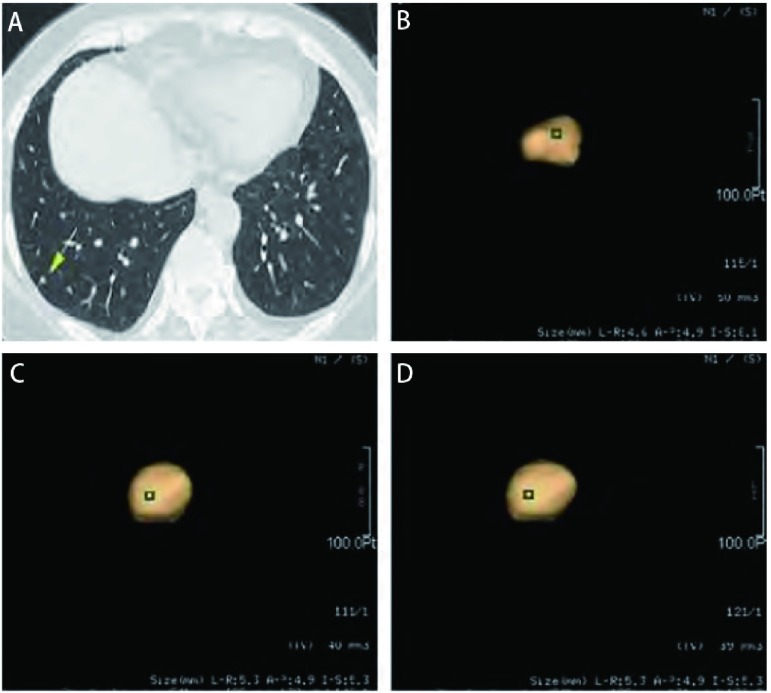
女，57岁。A：CT横断面显示右肺下叶小结节（箭），直径约4 mm；B-D：应用骨算法，不同层厚时所得结节容积；B：2.5 mm层厚，结节容积50 mm^3^；C：1.25 mm层厚，结节容积40 mm^3^；D：0.625 mm层厚，结节容积39 mm^3^。 Female, 57 years old. A: CT showed a 4 mm nodule in right lower lobe; B-D: The measured volumes using bone algorithm and different slice thickness; B: the volume is 50 mm^3^ when slice thickness is 2.5 mm; C: the volume is 40 mm^3^ when slice thickness is 1.25 mm; D: the volume is 39 mm^3^ when slice thickness is 0.625 mm.
